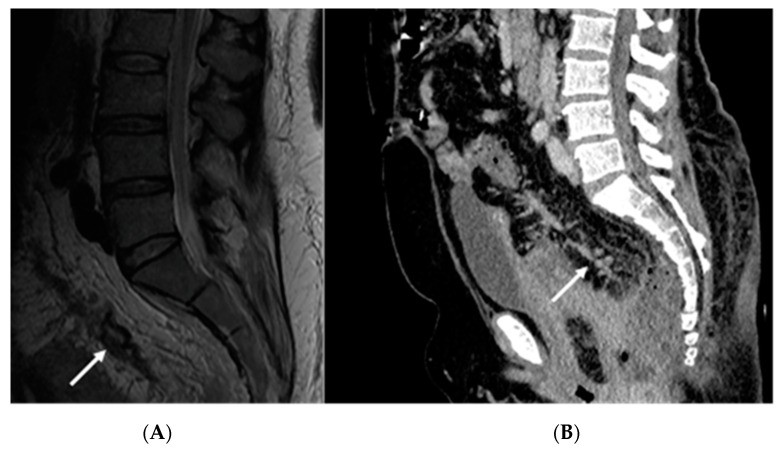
Sagittal T2 MRI (A) and sagittal CT image (B) depicting vascular invasion of the rectal tumor (white arrows).
